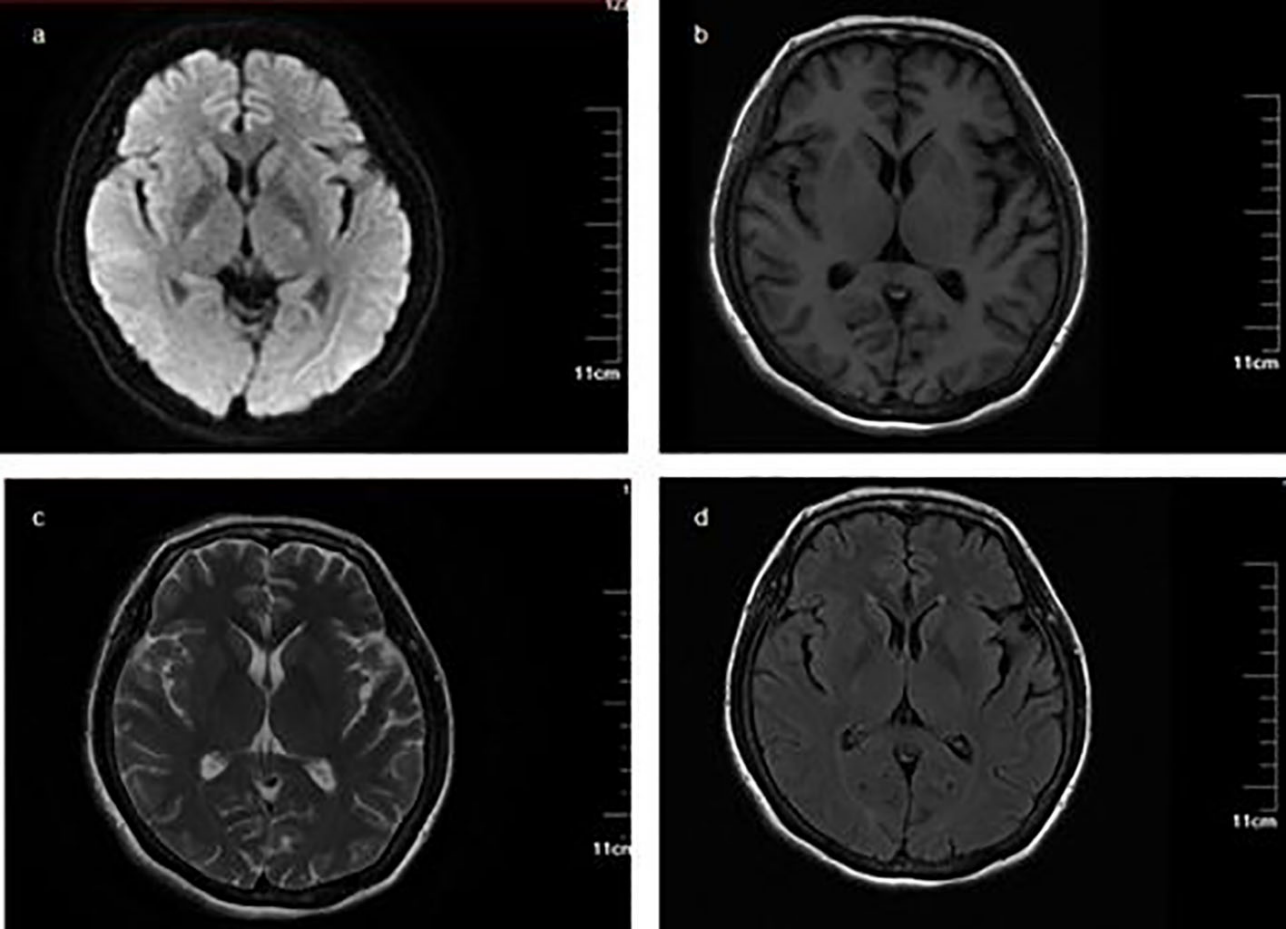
Magnetic resonance imaging and magnetic resonance angiography (MRA) MRI findings were unremarkable. (A) Diffusion-weighted imaging (DWI) sequence; (B) T1-weighted imaging; (C) T2-weighted imaging; (D) fluid-attenuated inversion recovery.
